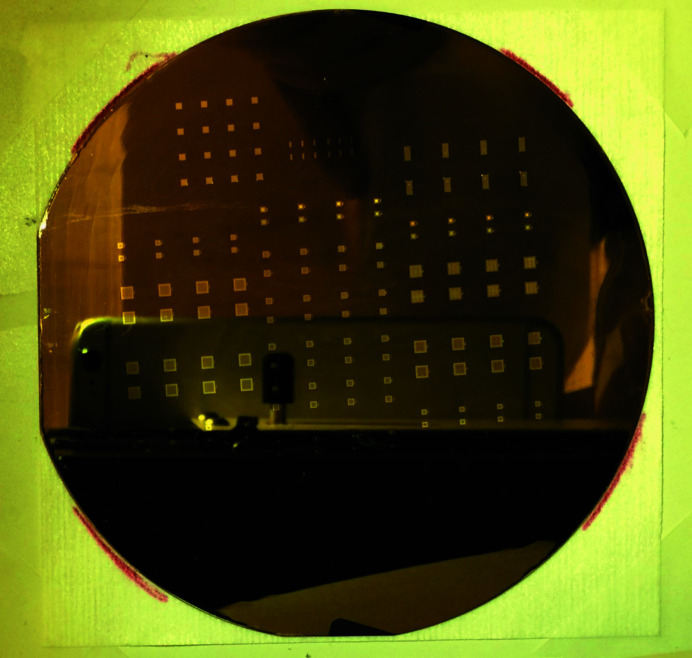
The finished silicon wafer prepared using the LIGA technique at the X-ray lithography facility at CAMD. It holds MURA of order 19, 37 and 73 as well as other objects such as single pinholes and slits.
